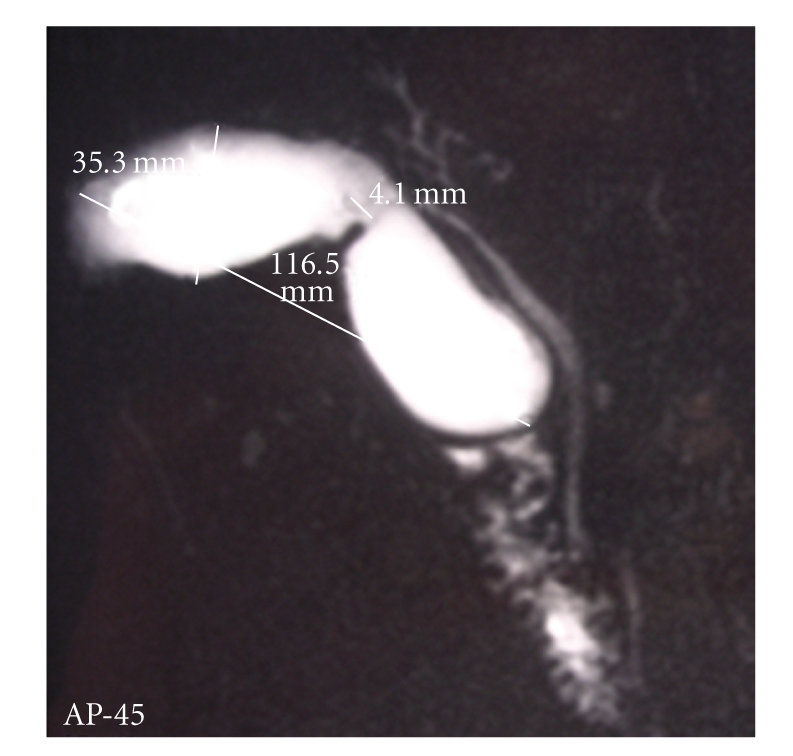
MRI findings. The cystic duct was not seen.
